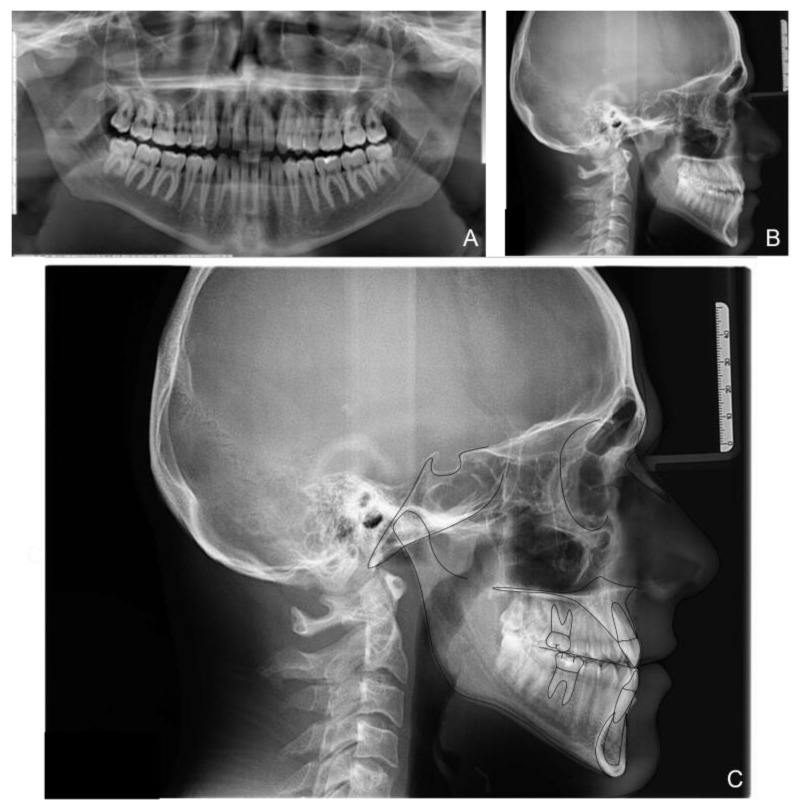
Patient X-rays, cephalometry tracing and analysis before treatment: panoramic X-ray (A); latero-lateral X-ray of the skull before treatment (B); Cephalometric tracing (C).
